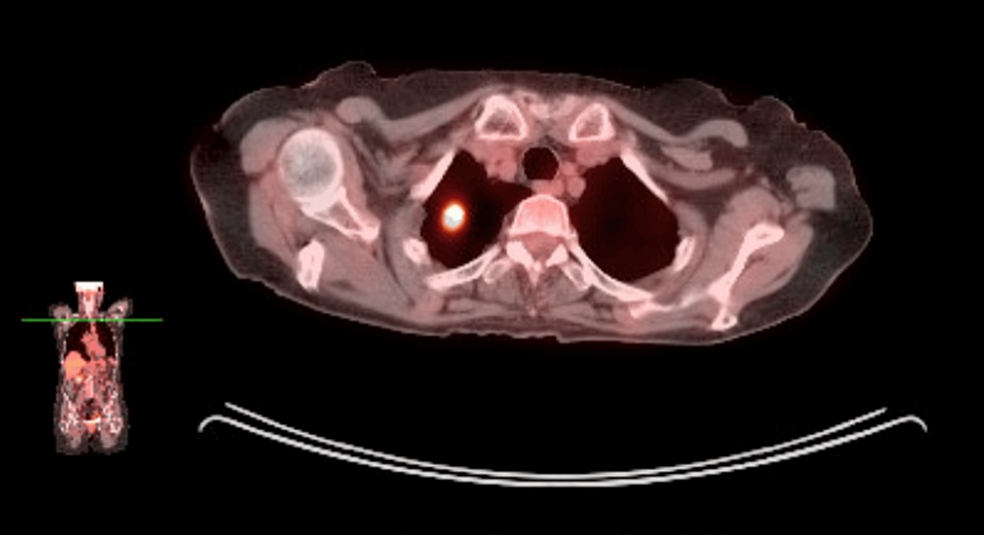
Whole body PET scan utilizing 16.831 mCi of F18-FDG demonstrates a 1.7 cm noncalcified, solid, speculated nodule in the right apical lung region, exhibiting high-grade metabolic activity with a maximum SUV of 12.9, set against a backdrop of surrounding pulmonary emphysema. SUV:  standardized uptake value; FDG: fluorodeoxyglucose
